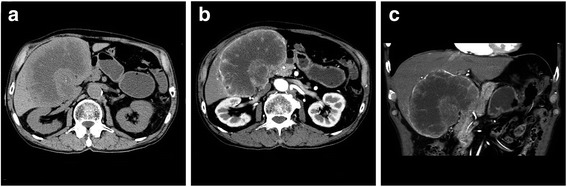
Axial abdominal CT. Large poorly marinated lobulated mass (a) with a heterogenous contrast enhancement (b, c)
